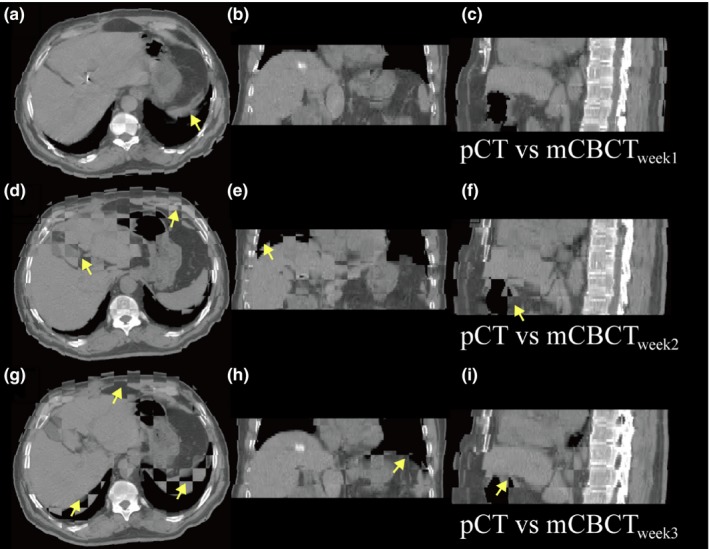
Checkerboard comparison between the pCT and mCBCT_weekn_ image before deformable registration, and the arrows indicate the visible differences.; (a), (b), and (c), the pCT vs mCBCT_week1_, (d), (e), and (f), pCT vs mCBCT_week2_, and (g), (h), and (i), pCT vs mCBCT_week3_.
